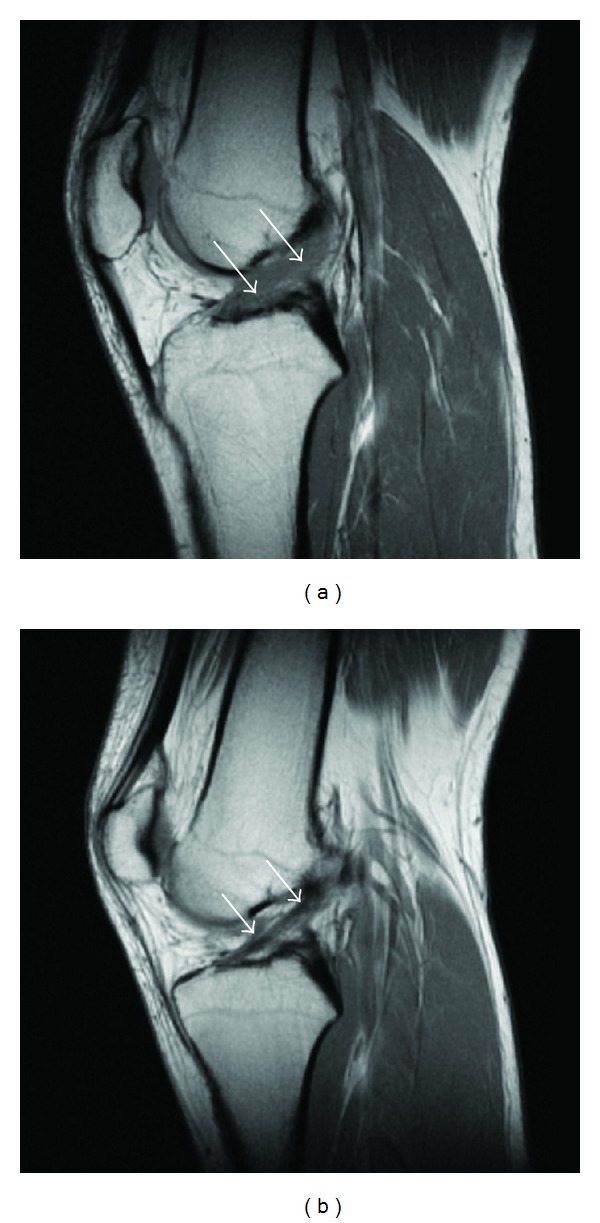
MRI. (a) MRI sagittal view of the knee shows a complete ACL rupture; the arrows point to where the ACL should be. (b) MRI sagittal view of the same patient after Manual Khalifa Therapy and 3 months of follow-up shows a continuous ACL; arrows are pointing out the “new” ACL.
